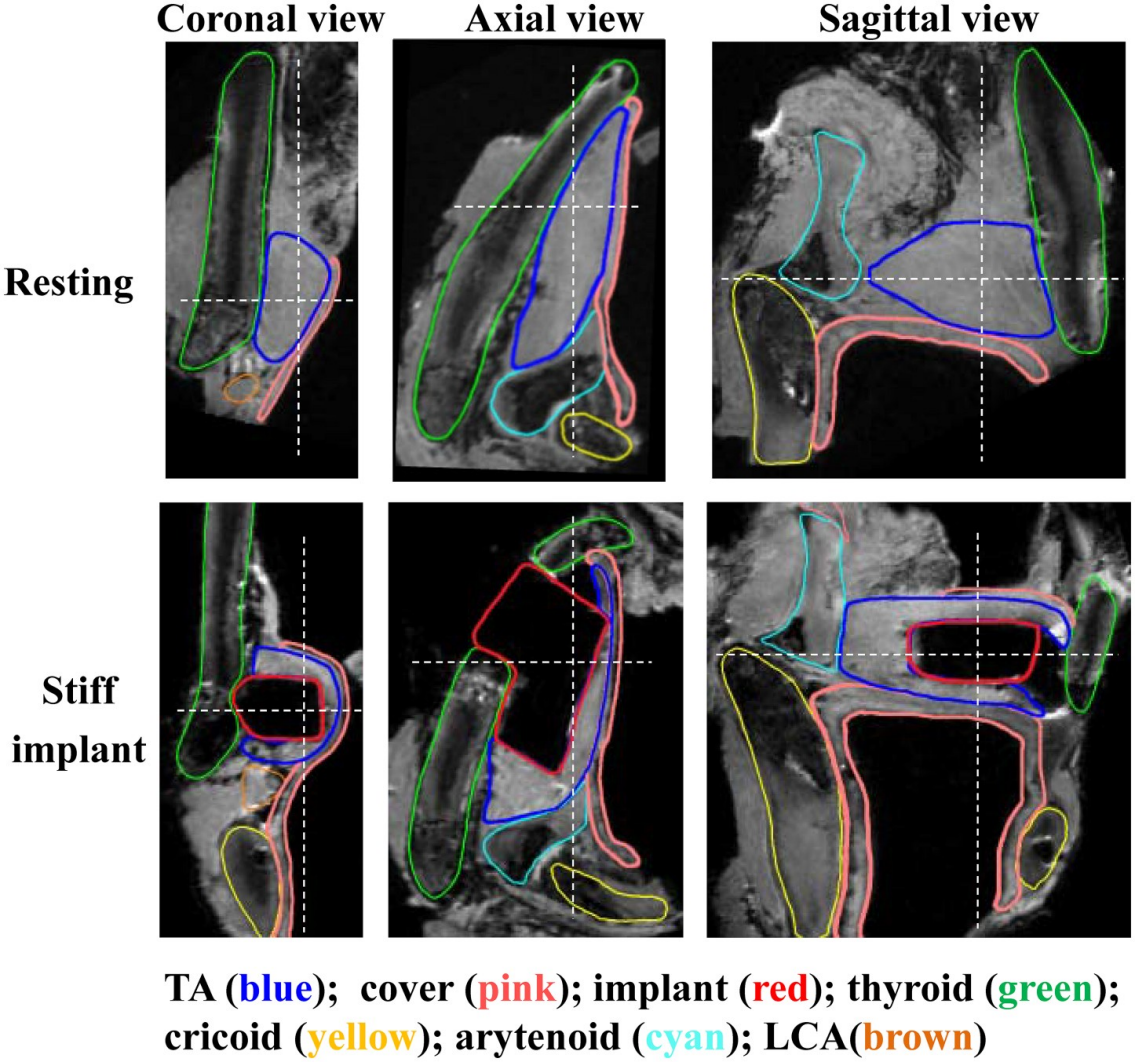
MRI images of larynx L2 (82 years old female) in three views. Top: the left half larynx at the resting position. Bottom: the right half with insertion of a stiff implant. The dashed white lines in each view indicate the cut planes from which the other two views were generated.
